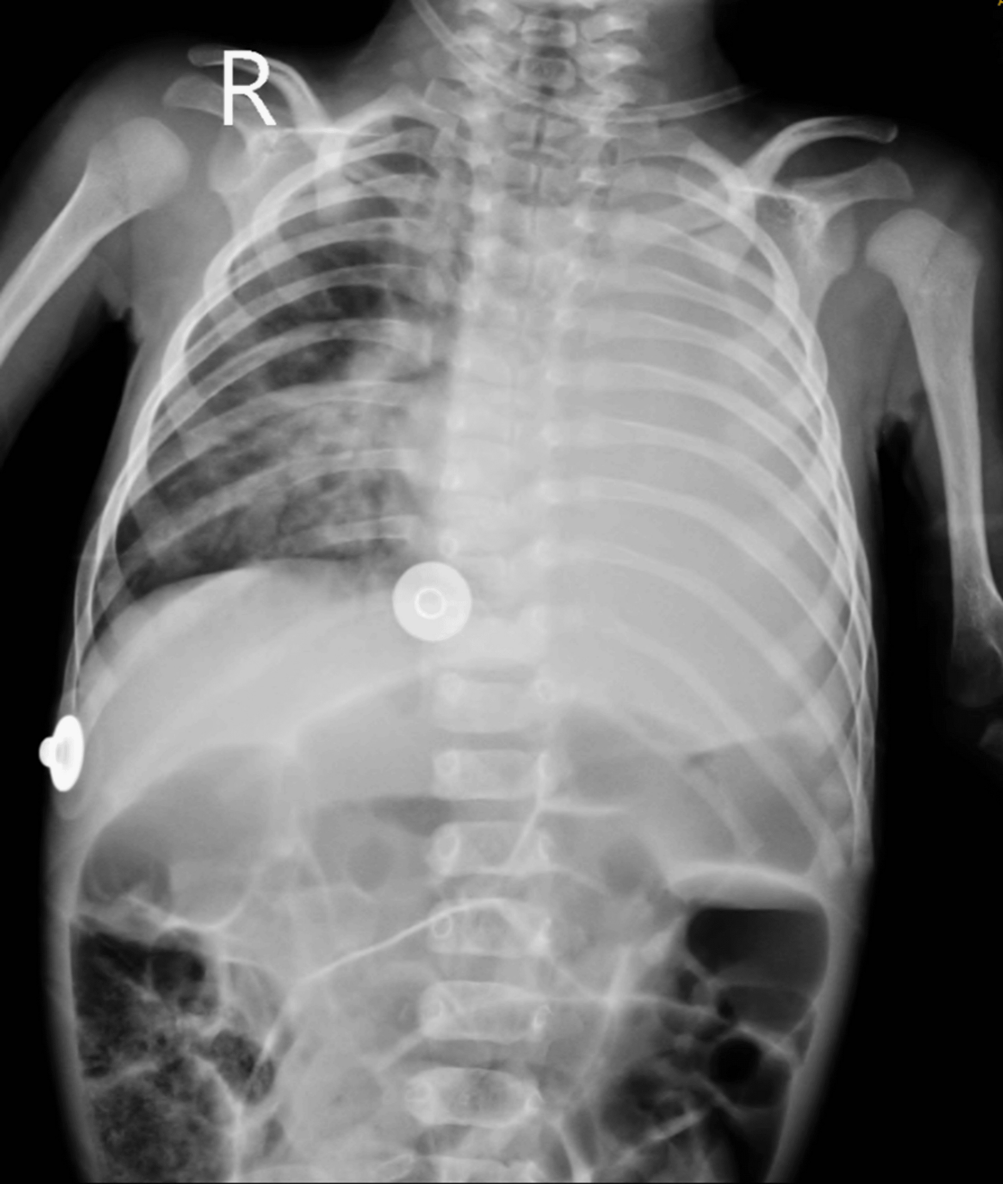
Pre-operative chest radiograph showing diffuse haziness in the left lung field
